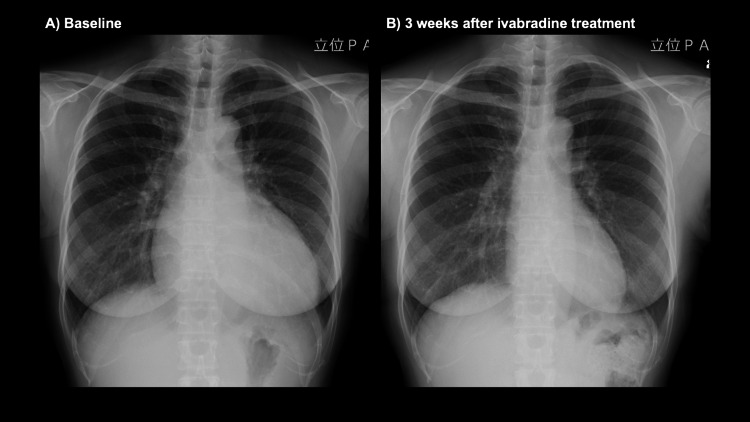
Chest X-ray before and after ivabradine treatment (A) Baseline. The chest-thoracic ratio was 58%. (B) Three weeks after ivabradine treatment. The chest-thoracic ratio was 42%.
